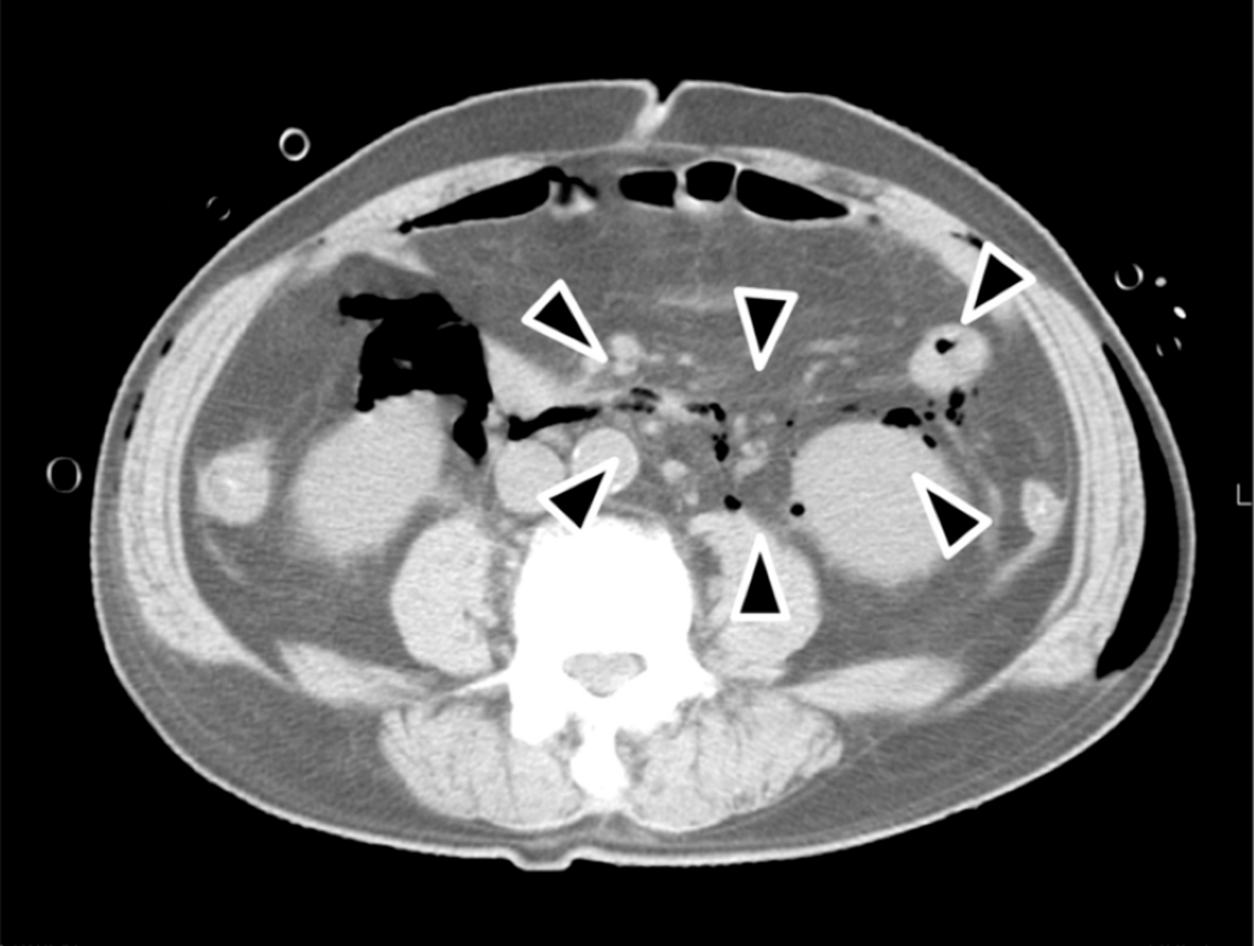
CT image under hospitalization of a patient (No.17) Axial CT image. Black arrows indicate the part of a gas production in the abdominal cavity.
